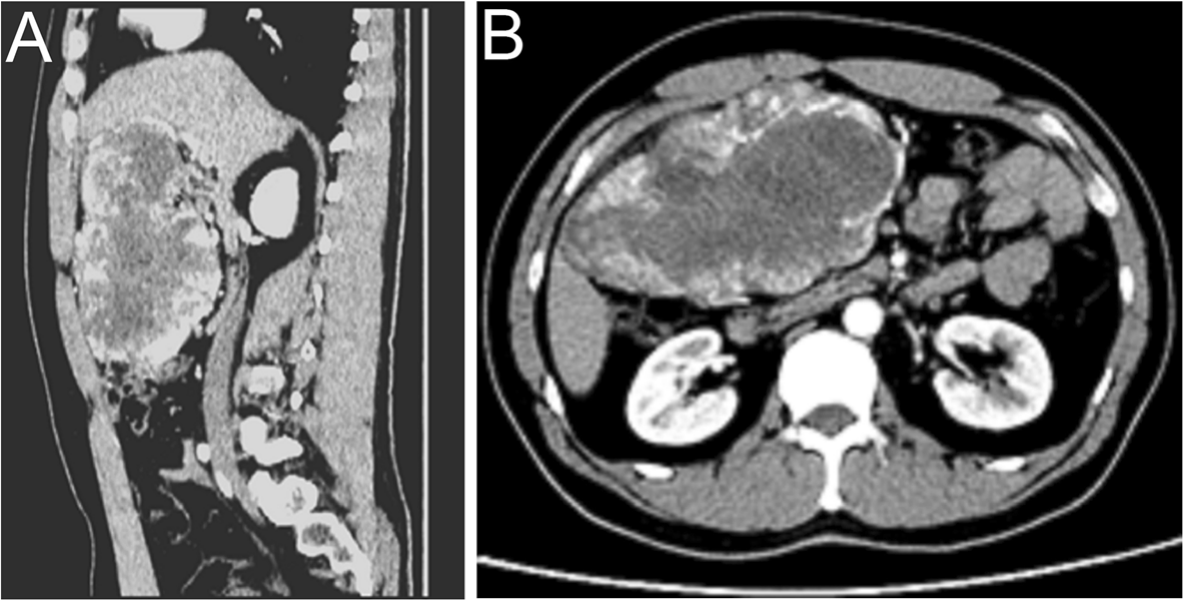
Abdominal CT scan shows a mass located in the right upper abdominal cavity and its three-dimensional reconstruction image. a: sagittal position, b: transverse position
